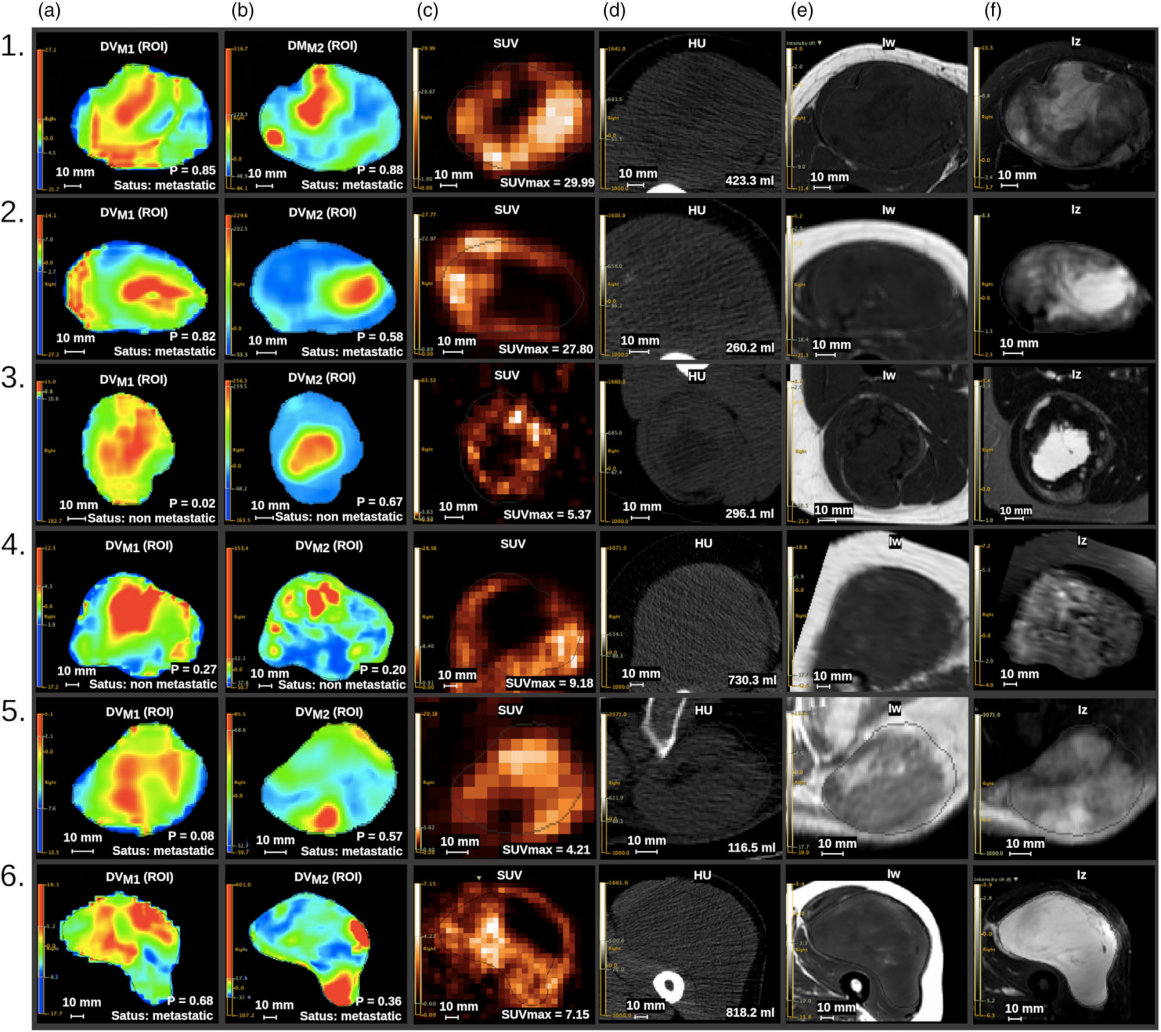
Slice examples of radiomic decision maps *D*V_M1_ (a) and *D*V_M2_ (b), positron emission tomography (PET) (c), computed tomography (CT) (d), T1 (e), and fat‐suppressed T2 (f) images for six patients
